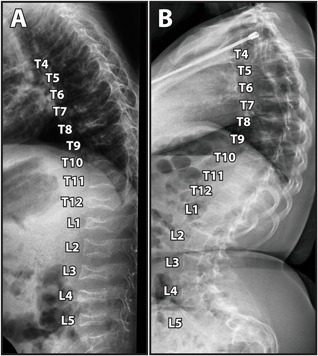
Lateral spine radiograph of a girl with osteogenesis imperfecta type IV showing reshaping of vertebral bodies during bisphosphonate treatment. (*A*) Age 2 years. (*B*) Age 15 years.
